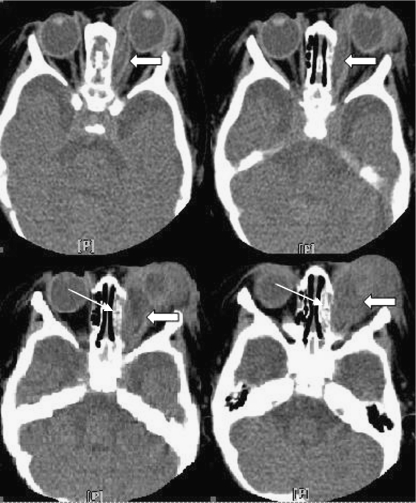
CT images demonstrated left ethmoid sinusitis (*thin white arrow*) and a left orbital abscess with adjacent soft tissue swelling (*thick white arrow*)
